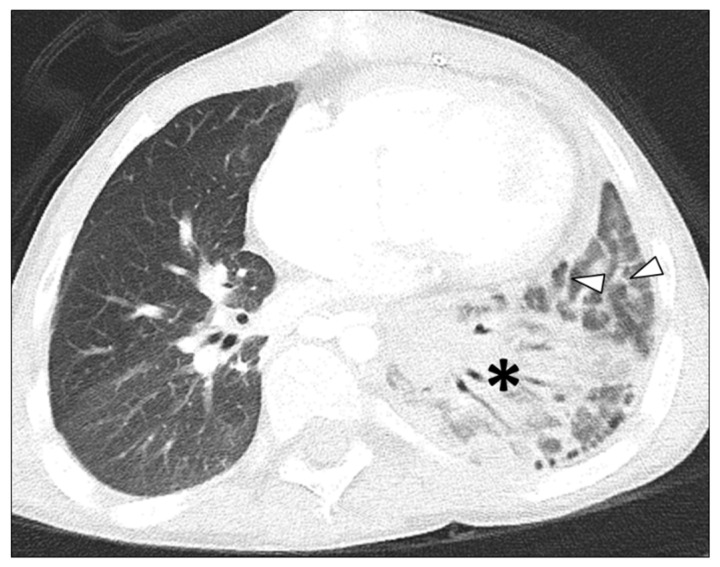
7-year-old male with left sided pulmonary vein stenosis and a history of aspiration. Axial lung window CT image demonstrates consolidation (asterisk) in the left lower lobe and septal thickening (arrowheads).
